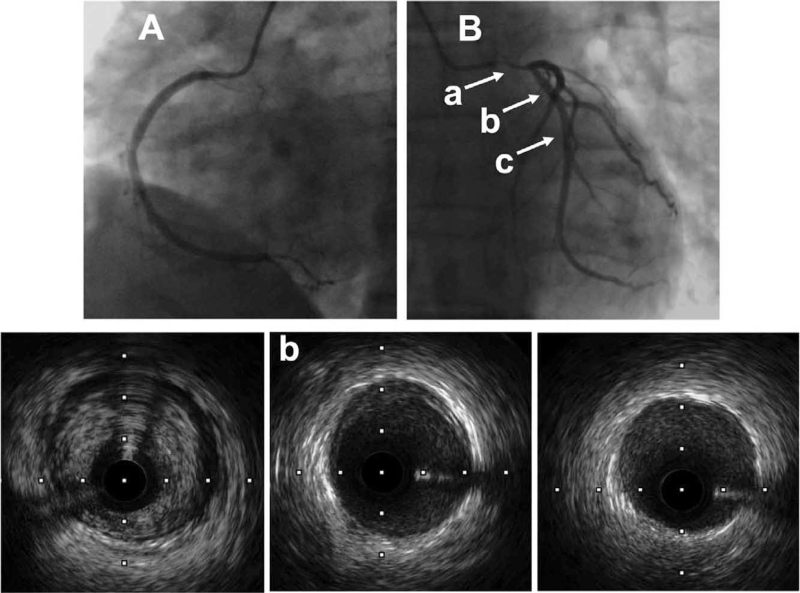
Urgent angiography shows a normal right coronary artery (A) and 80% to 90% stenosis of the left main coronary artery (B). The corresponding intravascular ultrasound cross-sectional image shows an eccentric homogenous fibrous plaque at the area of stenosis (a), and normal structure of the coronary artery distal to the lesion (b, c).
